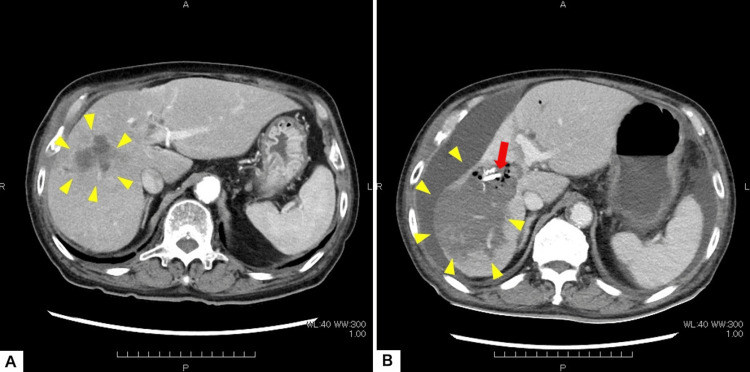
Progression of infectious complications. (A) Computed tomography (CT) showing initial formation of a liver abscess in the left lobe (arrowheads). (B) Terminal CT showing massive expansion of the abscess (arrowheads) despite drainage with a biliary stent (arrow).
